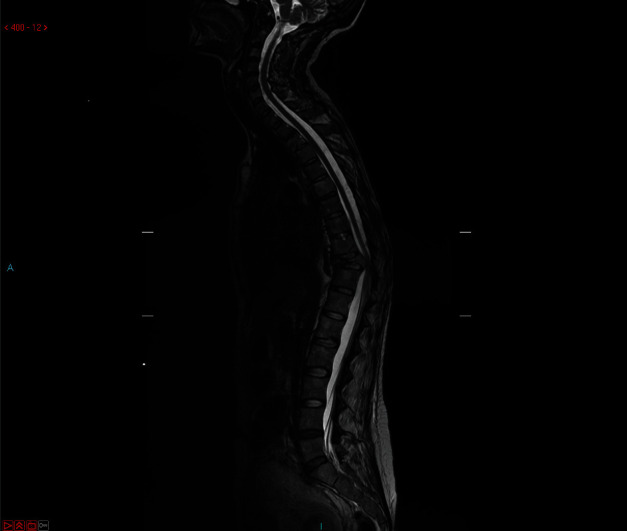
Preoperative MRI T2WI (whole spine).
